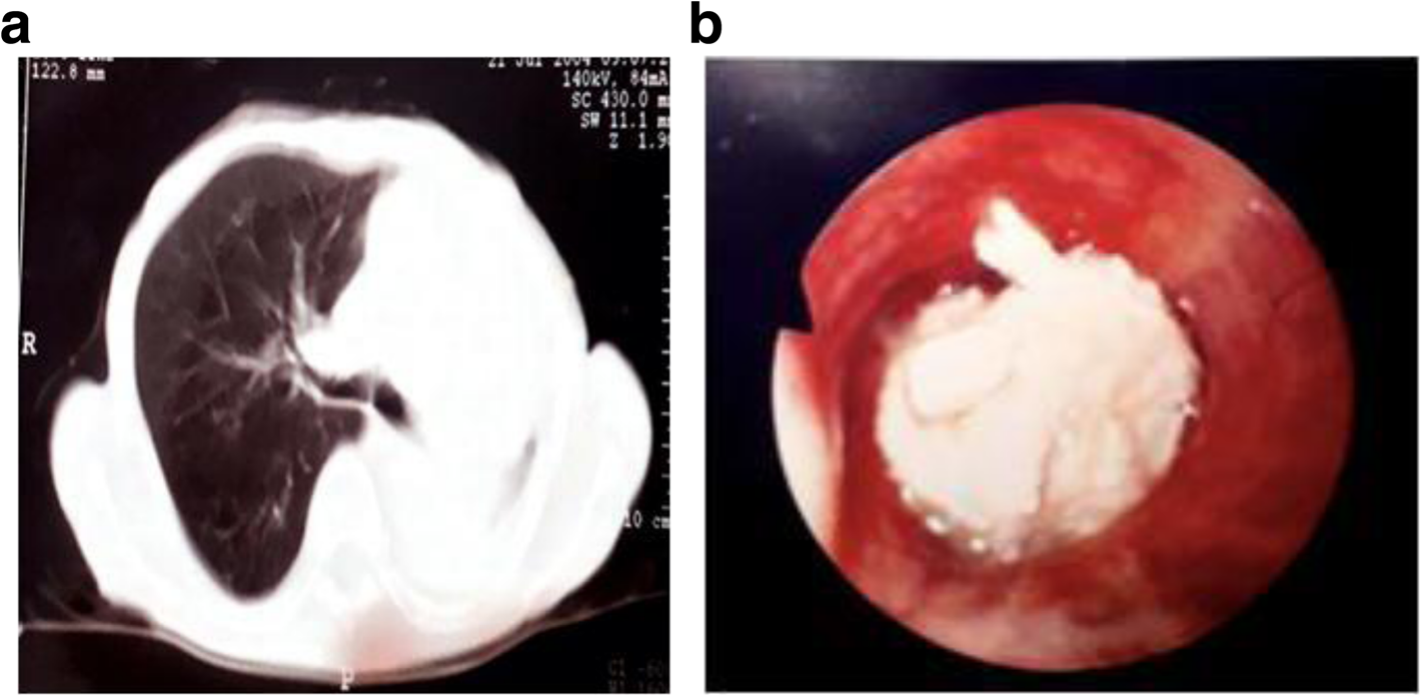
a Chest CT scan revealing a complete left atelectasis by endobronchial mass. b Bronchoscopic finding demonstrating the presence of an endobronchial white necrotized mass causing full occlusion of left main bronchus
